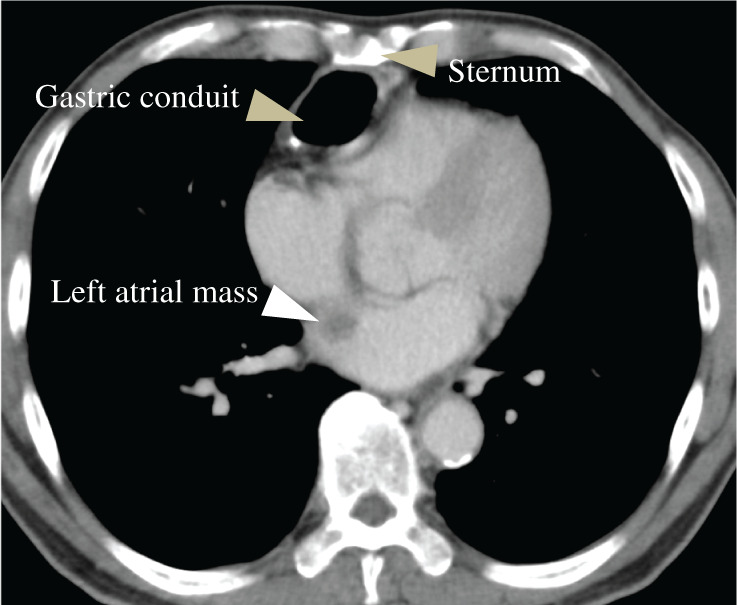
Contrast-enhanced CT showing a retrosternal reconstructed gastric conduit adjacent to the sternum (gray arrows) and a sessile left atrial mass (white arrow).
